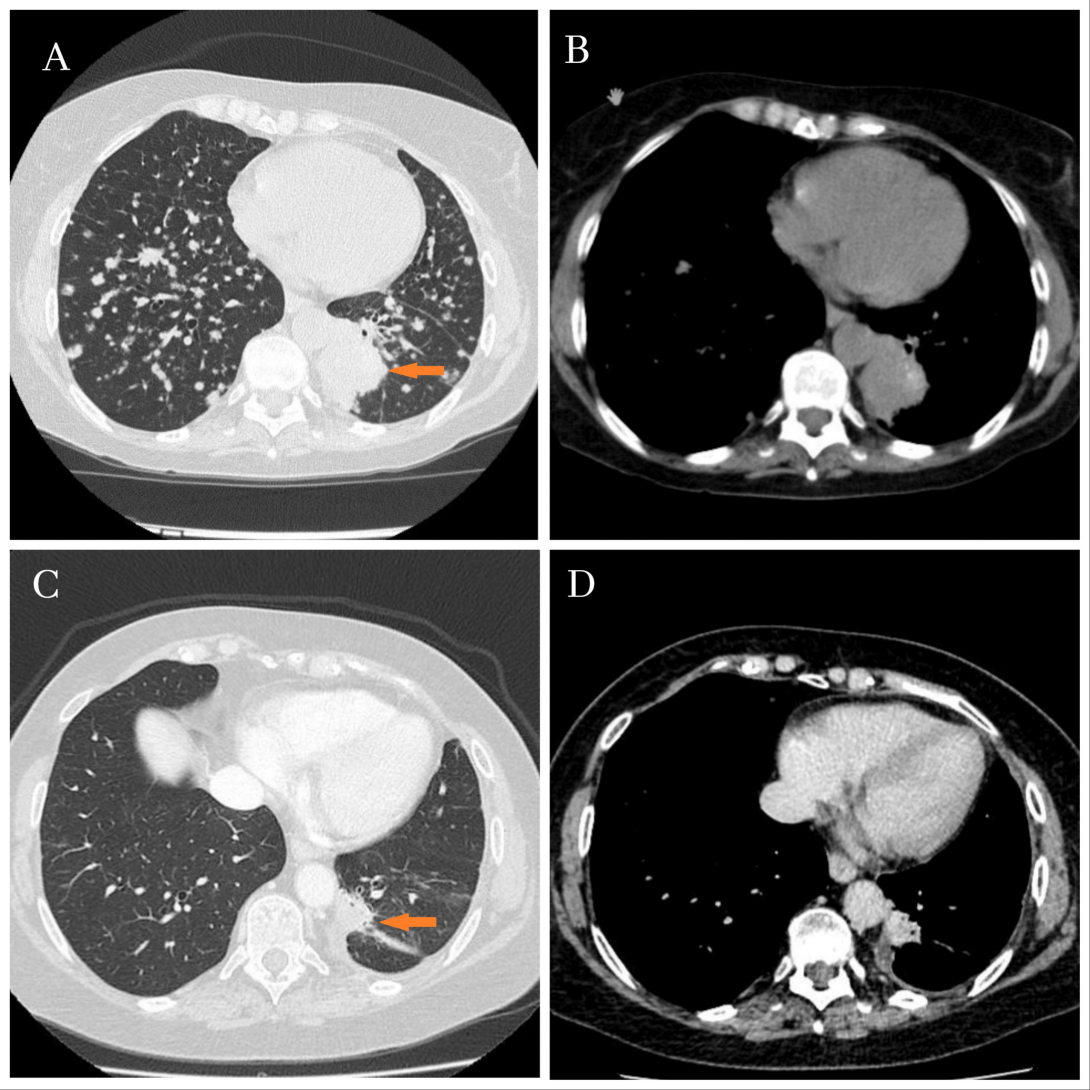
Thoracic computed tomography scan at diagnosis and after six cycles of the first-line therapy. Thoracic computed tomography scan at diagnosis, with a left paravertebral mass of 45 mm (arrow in A) and multiple millimetric nodules that were assumed as metastasis in both lungs (A and B). After six cycles of first-line therapy, a partial tumor response was observed, characterized by a significant reduction in the primary tumor size (arrow in C) and near-complete regression of bilateral micronodules (C and D).
